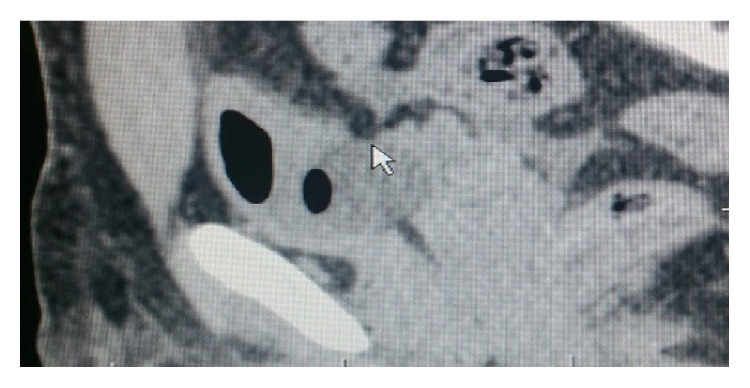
Plain CT scan showing the rent in the bladder marked by the arrow.
